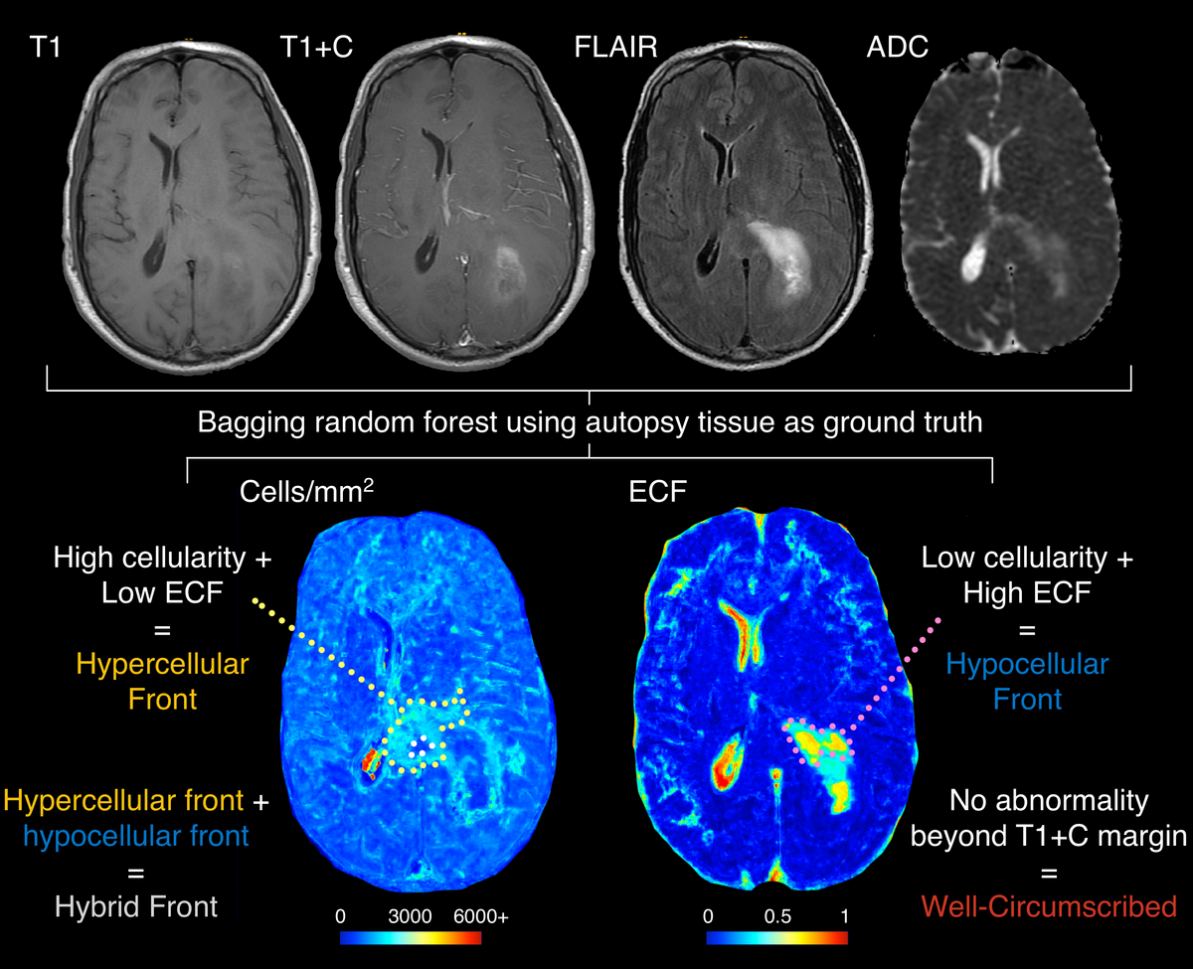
Overview of the study methodology. T1, T1+C, FLAIR, and ADC images were used to generate radio-pathomic maps of cell density (in cells/mm^2^) and extracellular fluid density (ECF, ranging from 0 to 1). These maps were then graded based on the presence of pathological abnormalities occurring outside the contrast enhancing region where hypercellular front patients exhibited high cell density and low ECF outside contrast, hypocellular front patients exhibited low cell density and high ECF outside contrast, hybrid front patients showed both hypercellular and hypocellular front regions (as seen in this example), and well-circumscribed patients showed no abnormal pathology beyond contrast enhancement.
